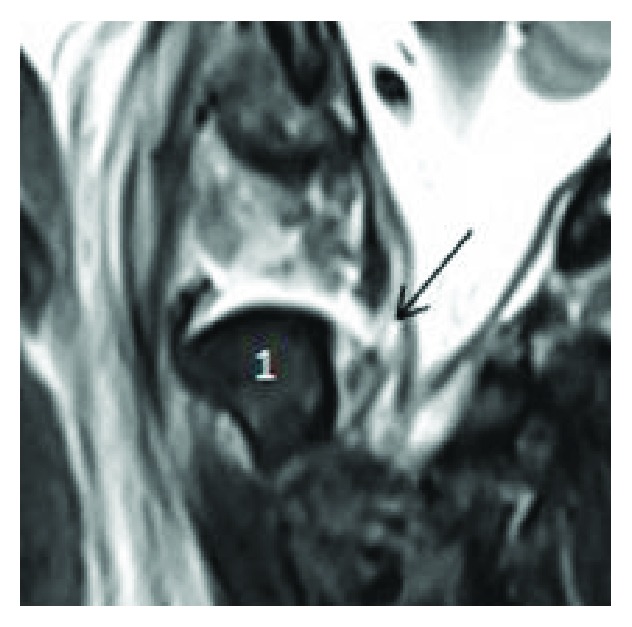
Cervical MRI sagittal view showing the tectorial membrane displaced off the dens (1) and a focal disruption of the left aspect (arrow). Note the prevertebral edema.
